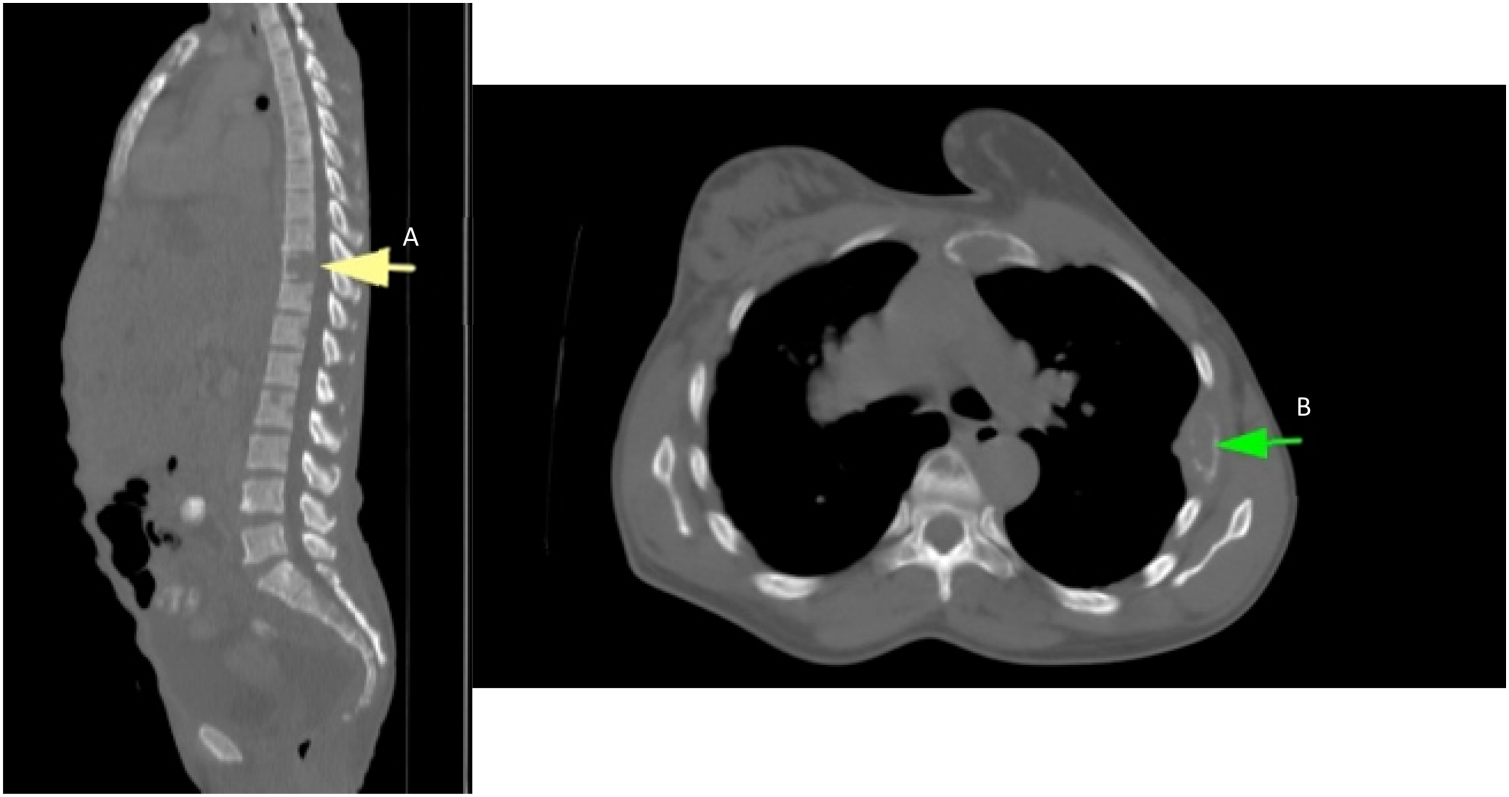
Sagittal (A) and axial (B) computed tomography scan images of the spine and chest, bone windows, showing lytic lesions in body of T10 vertebra and left rib (arrows).
